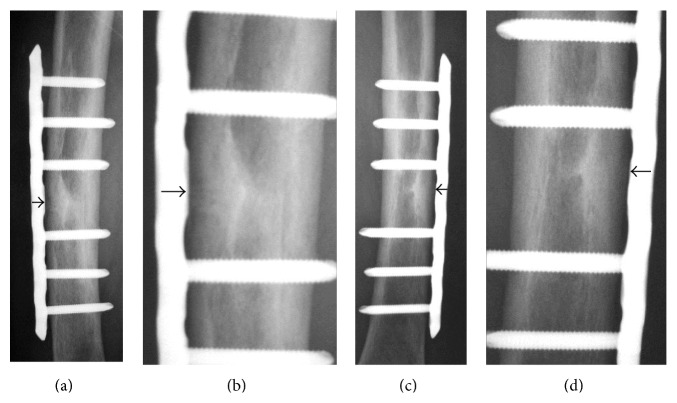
Anteroposterior radiographs of the bilateral femurs ((a) right femur; (c) left femur) 6 months after surgical treatment. (b) and (d) are magnified views of (a) and (c), respectively. The horizontal lines over the outer cortical bone (black arrows) had almost disappeared.
